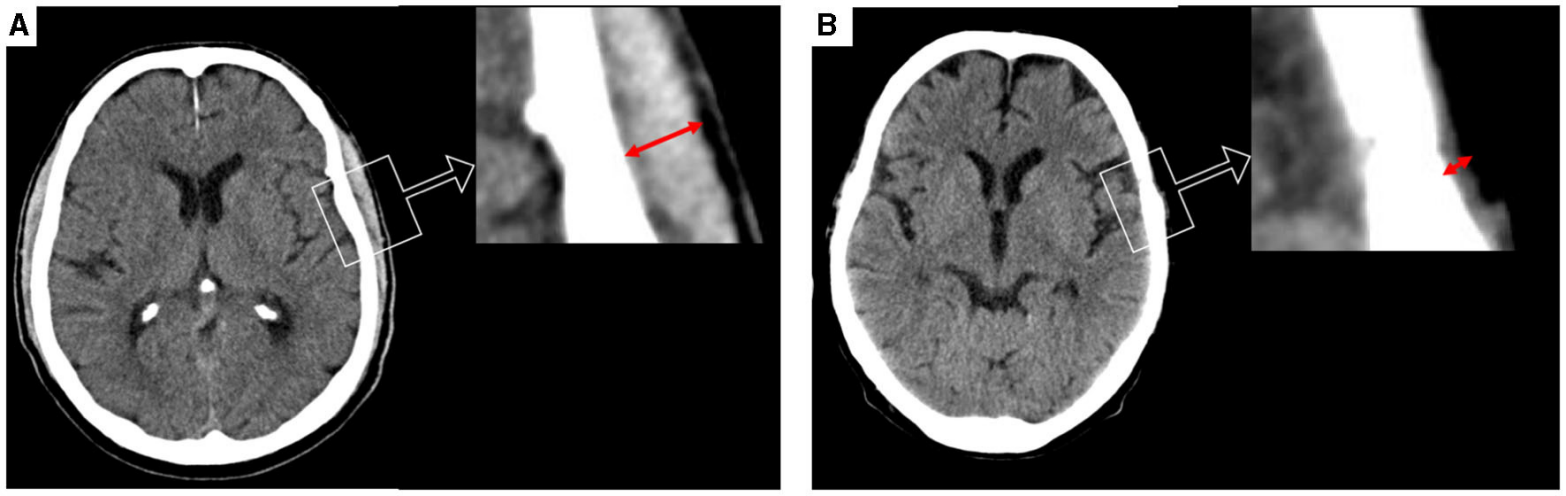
Representative cases for the assessment of temporalis muscle thickness (TMT) on cranial CT scan shown in red arrows. (A) TMT measurement on axial images of a patient with high TMT (bilateral median TMT = 10.5 mm), and (B) a patient with a low TMT on axial CT scan analysis (bilateral median TMT = 1.5).
